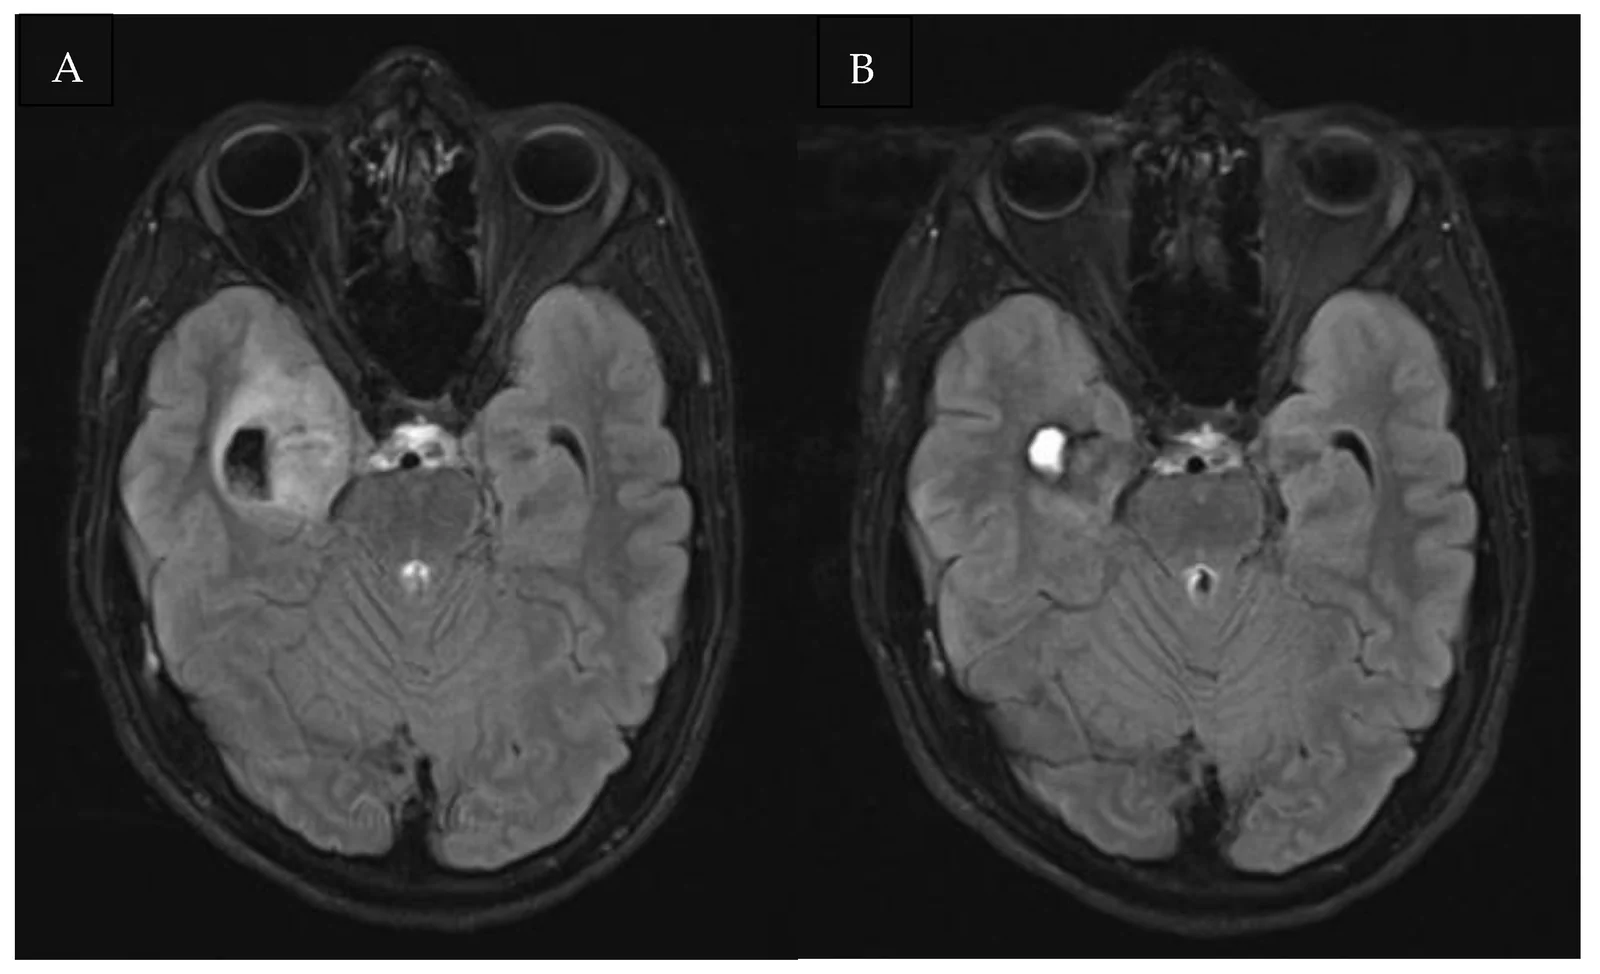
(A) Axial T2-weighted brain MRI obtained before stereotactic biopsy and cHL diagnosis, showing a right temporal lobe hyperintense lesion with haemorrhagic components. (B) Follow-up brain MRI performed after two cycles of ABVD during early response assessment.
